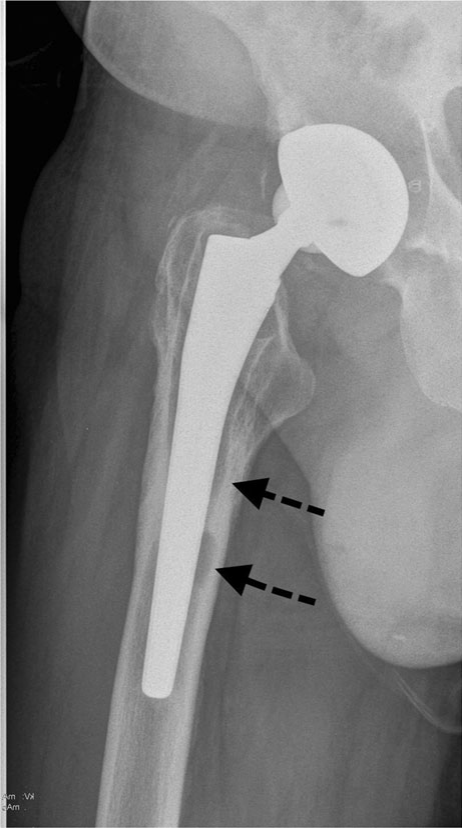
AP radiograph, 3 years postoperatively. Cementless bipolar hemiarthroplasty. Aseptic loosening, radiographically seen as periprosthetic lucencies in a Gruen zone 5/6 (*arrows*)
